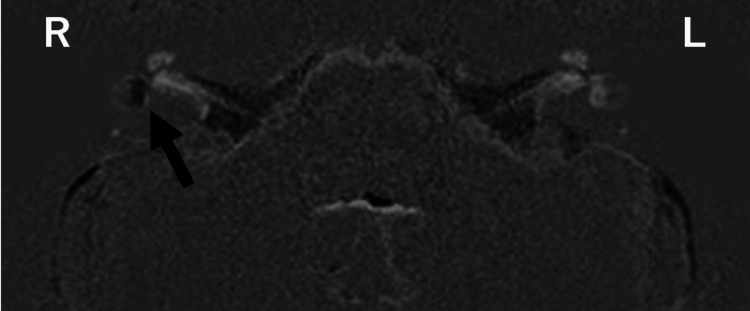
Marked endolymphatic hydrops in the right vestibule Following intravenous administration of a single dose of gadolinium-based contrast agent, inner ear MRI was performed using heavily T2-weighted cisternography, positive perilymph image (PPI), and positive endolymph image (PEI) sequences to visualize the endolymphatic space. No abnormal findings suggestive of endolymphatic hydrops were observed in either cochlea. However, the right vestibule showed marked endolymphatic hydrops occupying more than half of the entire vestibular area. No hydrops was detected in the left vestibule. No findings suggestive of neurovascular compression or nerve entrapment were observed.
